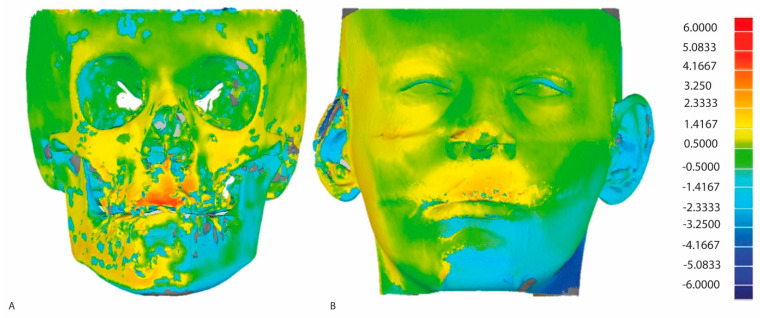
Colormaps of soft and hard tissues in the 3D surface models obtained using CBCT. (A) hard tissue colormap; (B) soft tissue colormap. The scale bar indicates the direction and mm of the displacement between T0 and T1.
